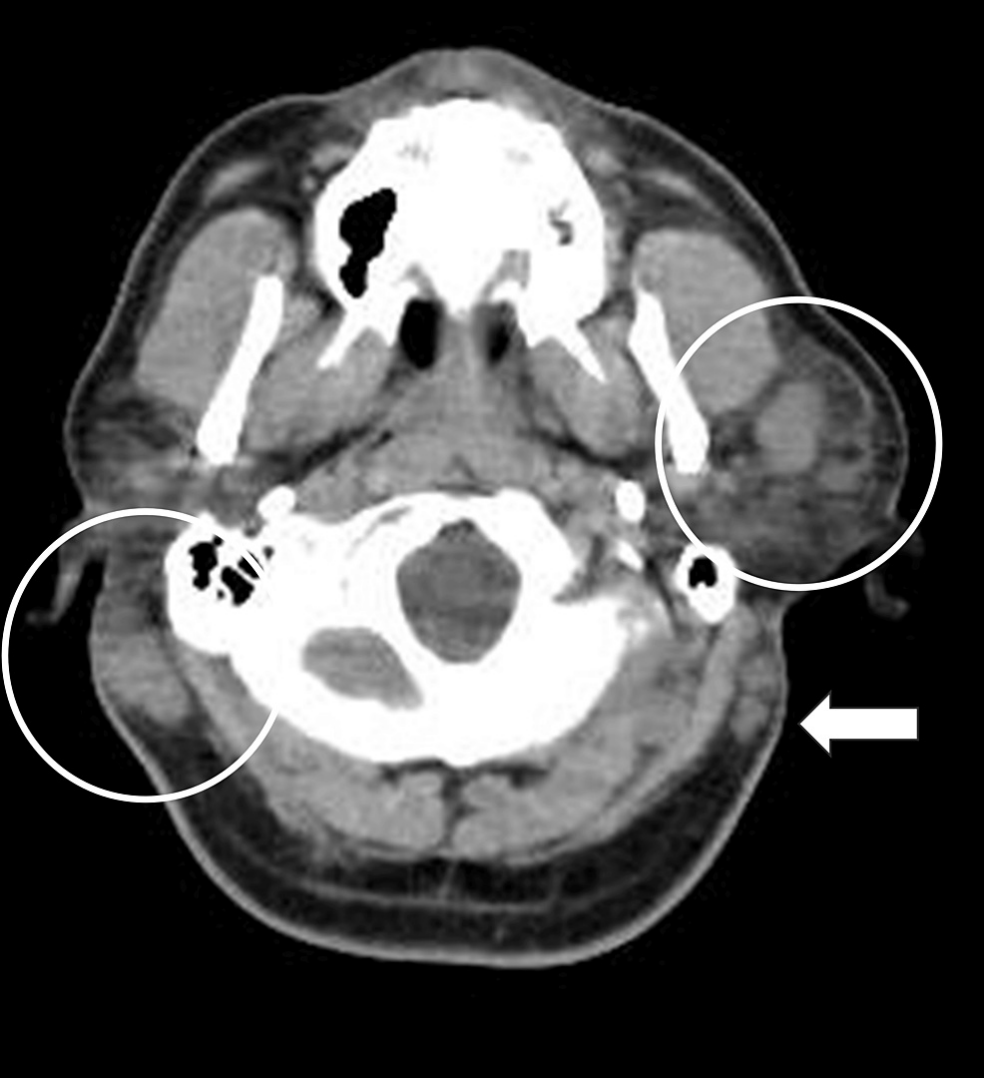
Cervical CT An infiltrative lesion with ill-defined borders has formed beneath the skin adjacent to the parotid gland (white circle). Enlarged lymph nodes are seen in the surrounding area (white arrows).
